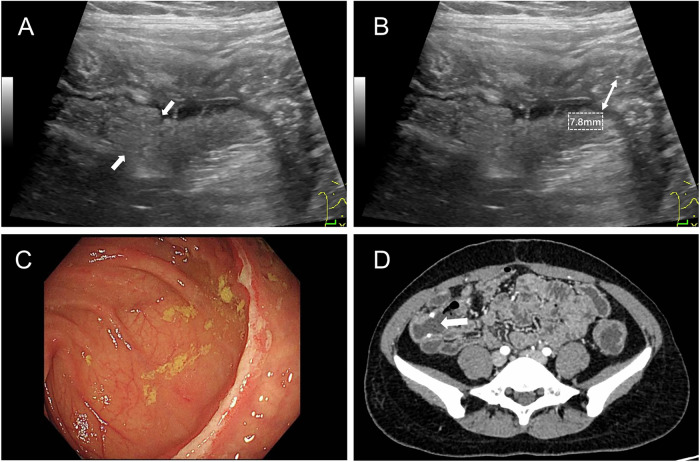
A 20-year-old female patient with CD exhibited thickening of the ileum with HMF (A, solid arrow). The thickness of the diseased bowel was measured at 7.8 mm (B). Eight months after an ileocecectomy, inflamed mucosa with ulcers was detected during endoscopy (C), and a CT scan showed that the bowel wall at the anastomosis (hollow arrow) was thickened (D). CD, Crohn’s disease; HMF, hyperechoic mesenteric fat
